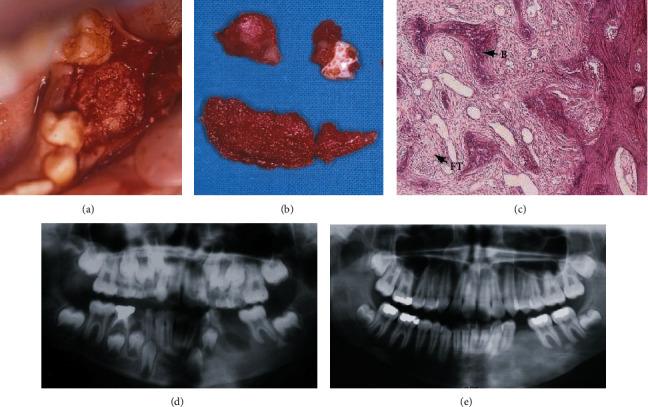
(a) Clinical view of the excess bone. (b) The excised bone with the dental germ. (c) HE × 10 showing irregularly shaped trabeculae of immature bone within proliferating fibroblastic tissue (B: bone; FT: fibrous tissue). (d) One-week panoramic radiograph. (e) Five-year panoramic radiograph.
